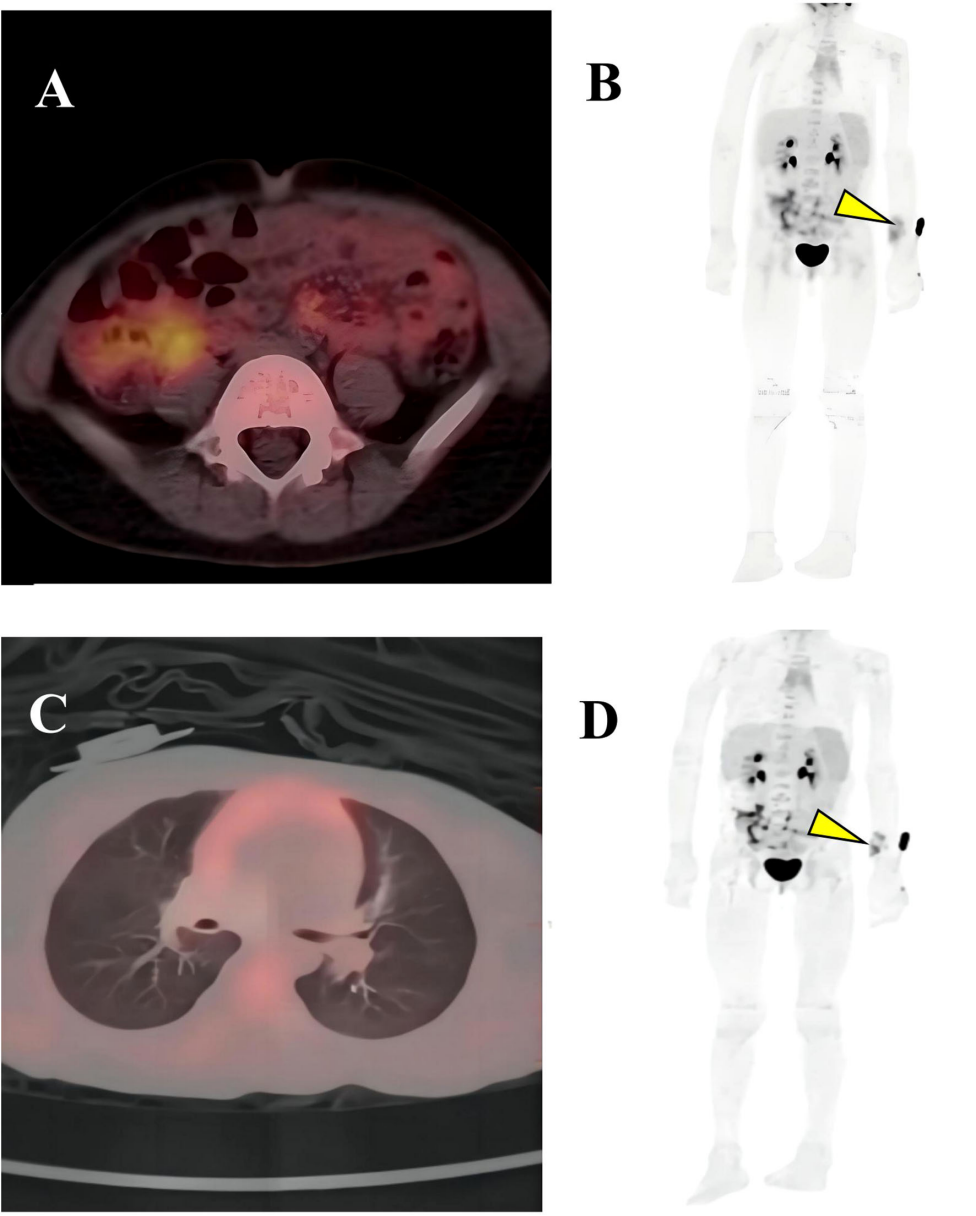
PET-CT imaging. (A, B) Showed soft tissue changes with increased FDG uptake; (C, D) Slightly elevated FDG uptake suggests residual tumor, but no systemic metastases are observed. Residual lesion in the left wrist (yellow arrow).
